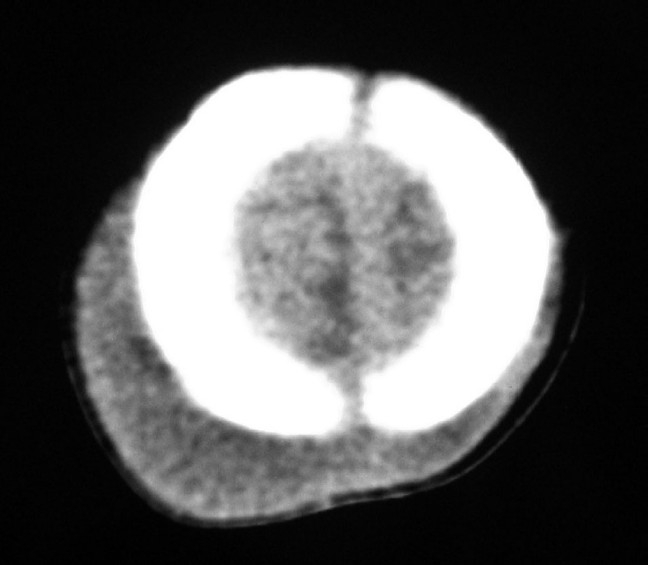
Non-contrast head CT of infant demonstrating soft tissue fluid collection. The Hounsfield units were consistent with water/ CSF. No fractures were identified
